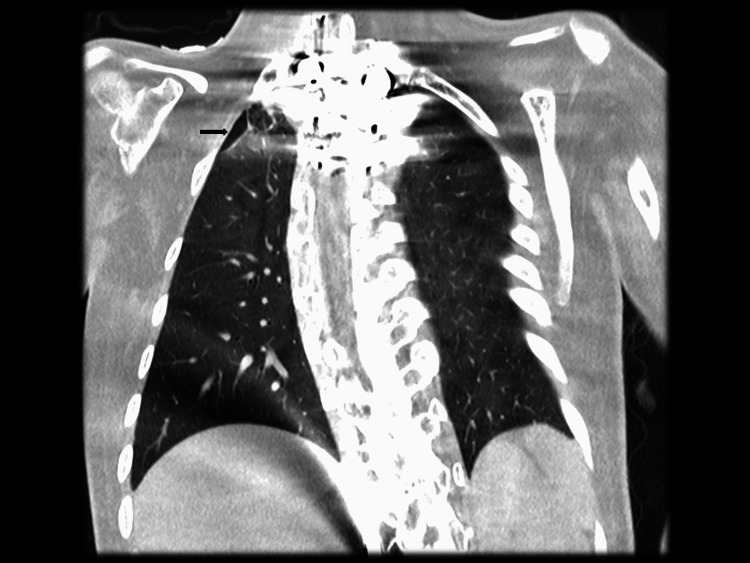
Coronal image of computed tomography scan showing partial resolution of right-side pneumothorax with residual pneumothorax on the right upper lobe (black arrow).
